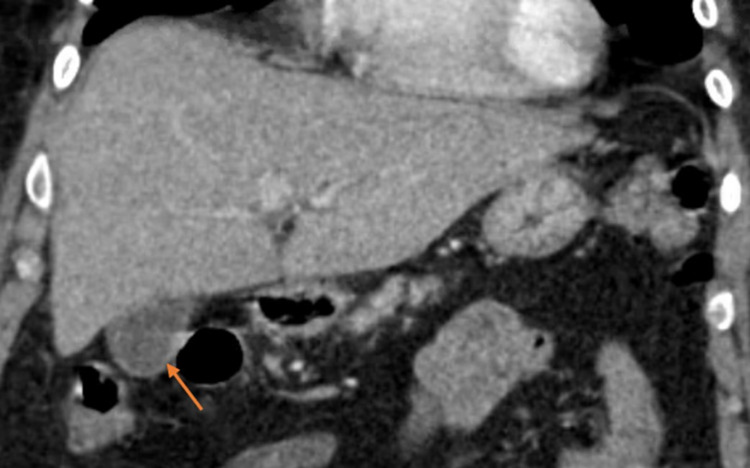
CT image demonstrating enhancing GB wall thickening (arrow) in the coronal plane with no evidence of gallstones CT - computed tomography; GB - gallbladder
